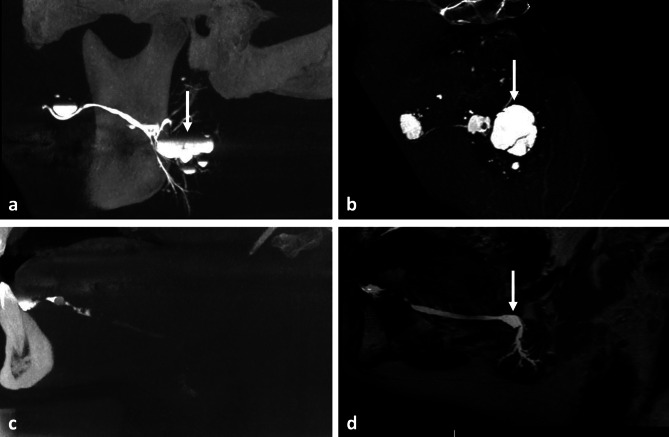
Exploration of salivary dilatations. Analysis of the left parotid gland revealing the presence of sialoceles of the anterior part of the main duct and in an intraglandular location (white arrow) with 3D-CBCT sialography in sagittal oblique MIP view (a) and MR sialography in native sagittal view (b). Case of a patient presenting with a distal obstructive sialolithiasis of the right wharton’s duct, with no upstream opacification in 3D-CBCT sialography in sagittal view (c), and with proximal dilatation of the salivary ducts visible on the MR sialography in MIP sagittal view (d)
